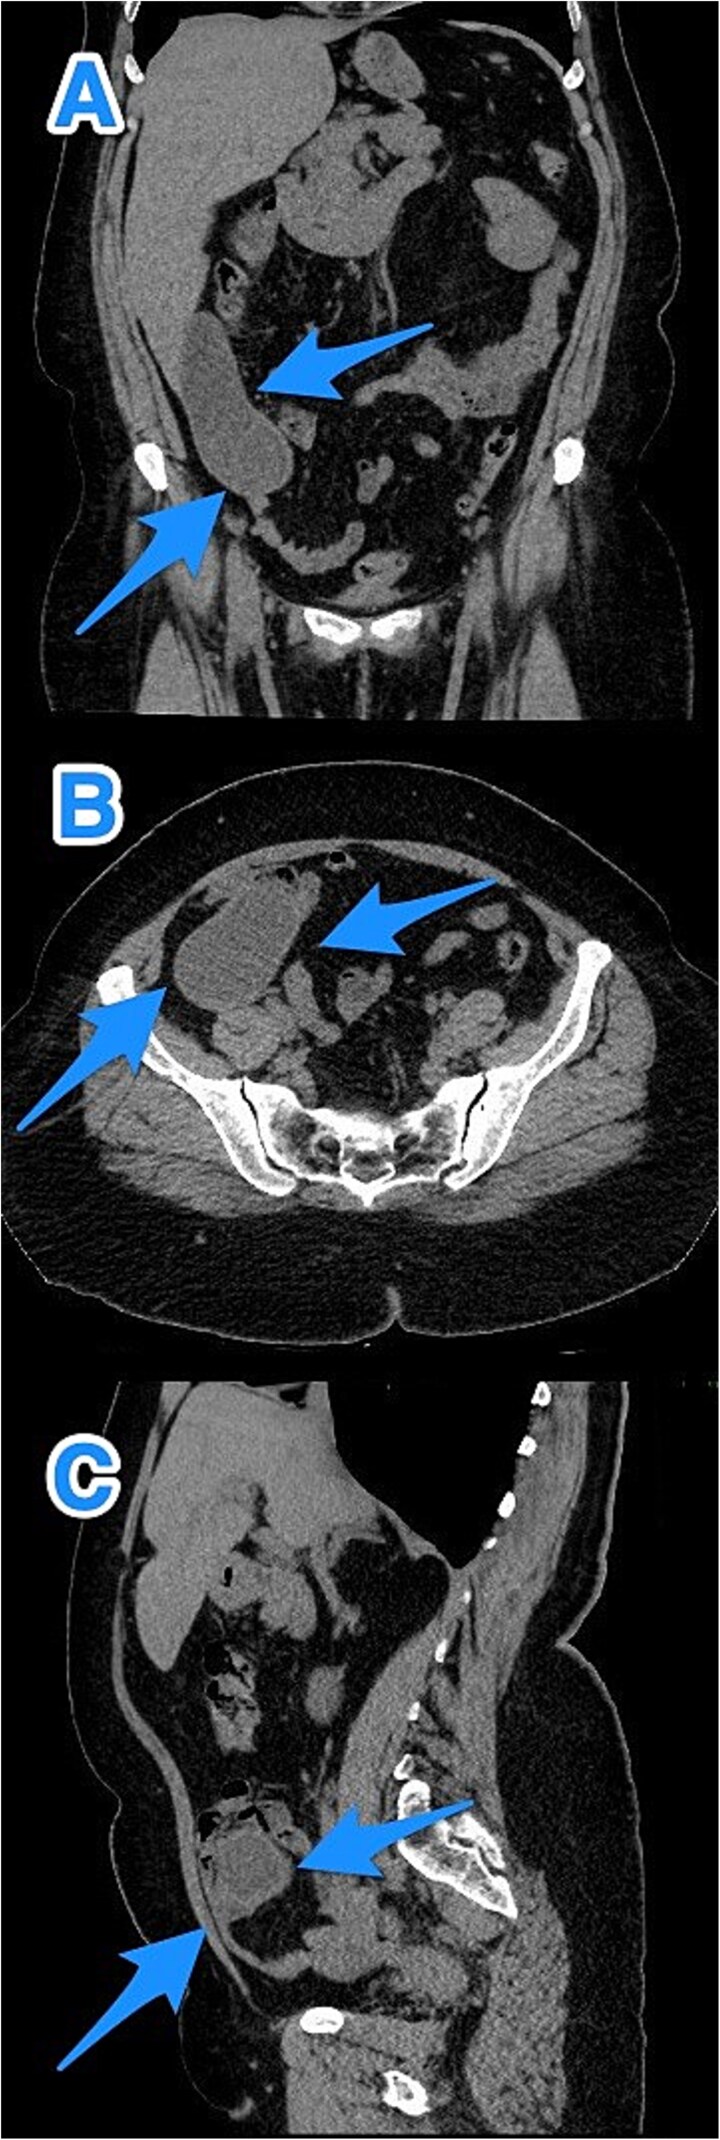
CT abdomen and pelvis with contrast: (A) coronal, (B) axial, and (C) sagittal views reveal an appendix measuring 22 cm in the right iliac fossa, with edematous wall thickening, minimal surrounding fat stranding, and an adjacent fluid collection measuring 3.3 × 1.8 × 8.5 cm, consistent with a perforated appendix (arrows).
